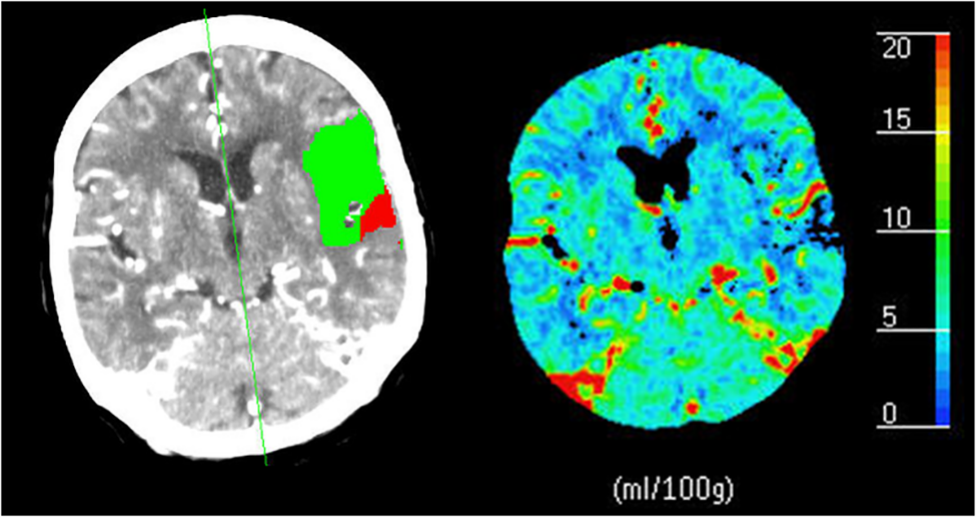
A typical CT perfusion summary map and blood volume map. An example of a thick slice (5 mm) CTP summary map (left) for acute stroke assessment of a patient with an occluded left middle cerebral artery. The red area indicates the infarct core and the green area indicates the penumbra (tissue at risk). The right parameter map shows the cerebral blood volume (CBV). Both maps were generated by the Philips EBW 4.0 Brain Perfusion software (Philips Healthcare, Best, The Netherlands). The thresholds for the infarct core and penumbra were respectively CBV < 2.0 mL/100g and MTT > 145% of the contralateral side, as suggested by Wintermark et al. [11].
